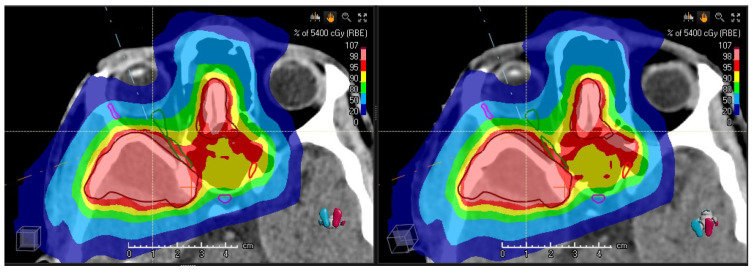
Clinical validation case. Left: Dose distribution of the initial treatment plan calculated and overlaid on the verification CT. Right: Dose distribution calculated an overlaid on the synthetic CT.
